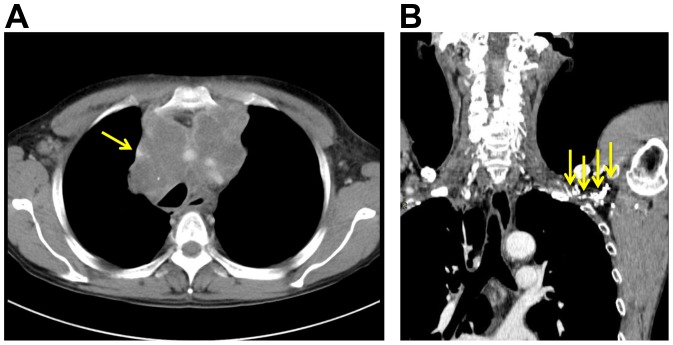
Examples of anticipated preoperative difficulties with the procedure confirmed on CT scan images. (A) SVC syndrome.
